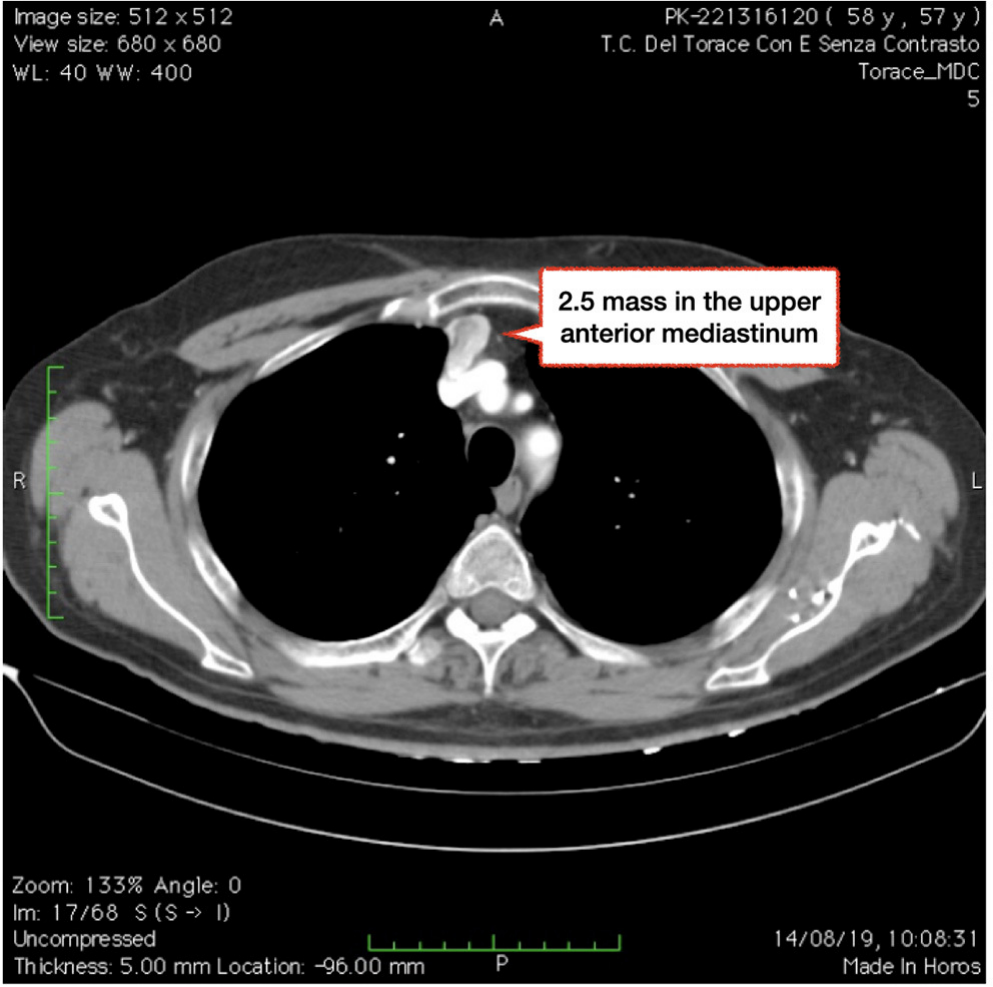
CT scan showing a 2.5 cm mass in the upper anterior mediastinum, with positive contrast enhancement.
